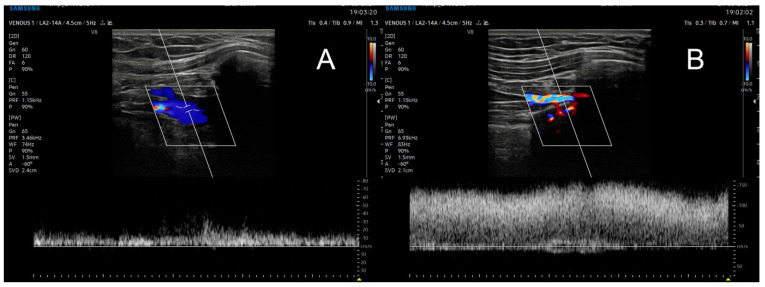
Ultrasound examination of the subclavian vein in resting position (A) and in abduction to 90 degrees (B), with a high increase in flow near occlusion.
